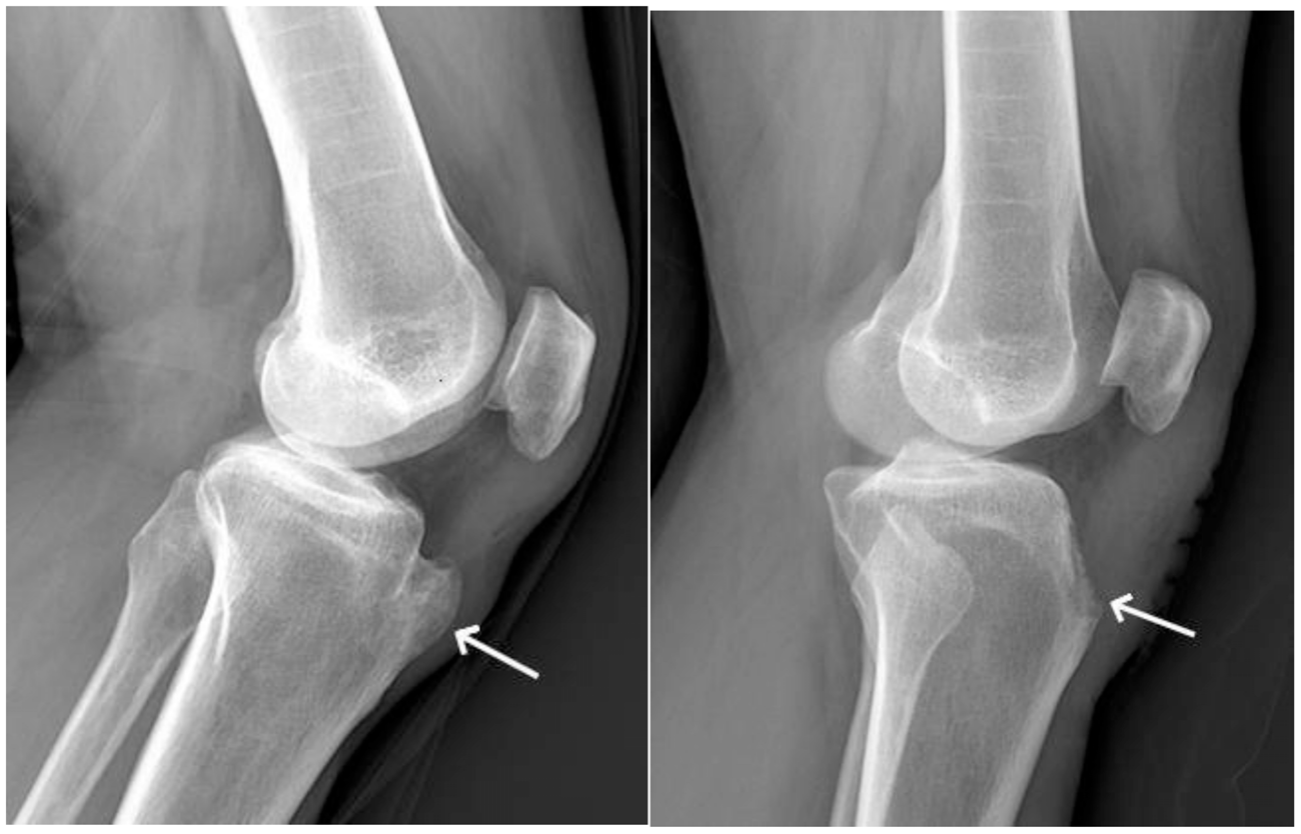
Preoperative radiographs of osteochondroma of the tibial tuberosity combined with gouty calculus (white arrows).
